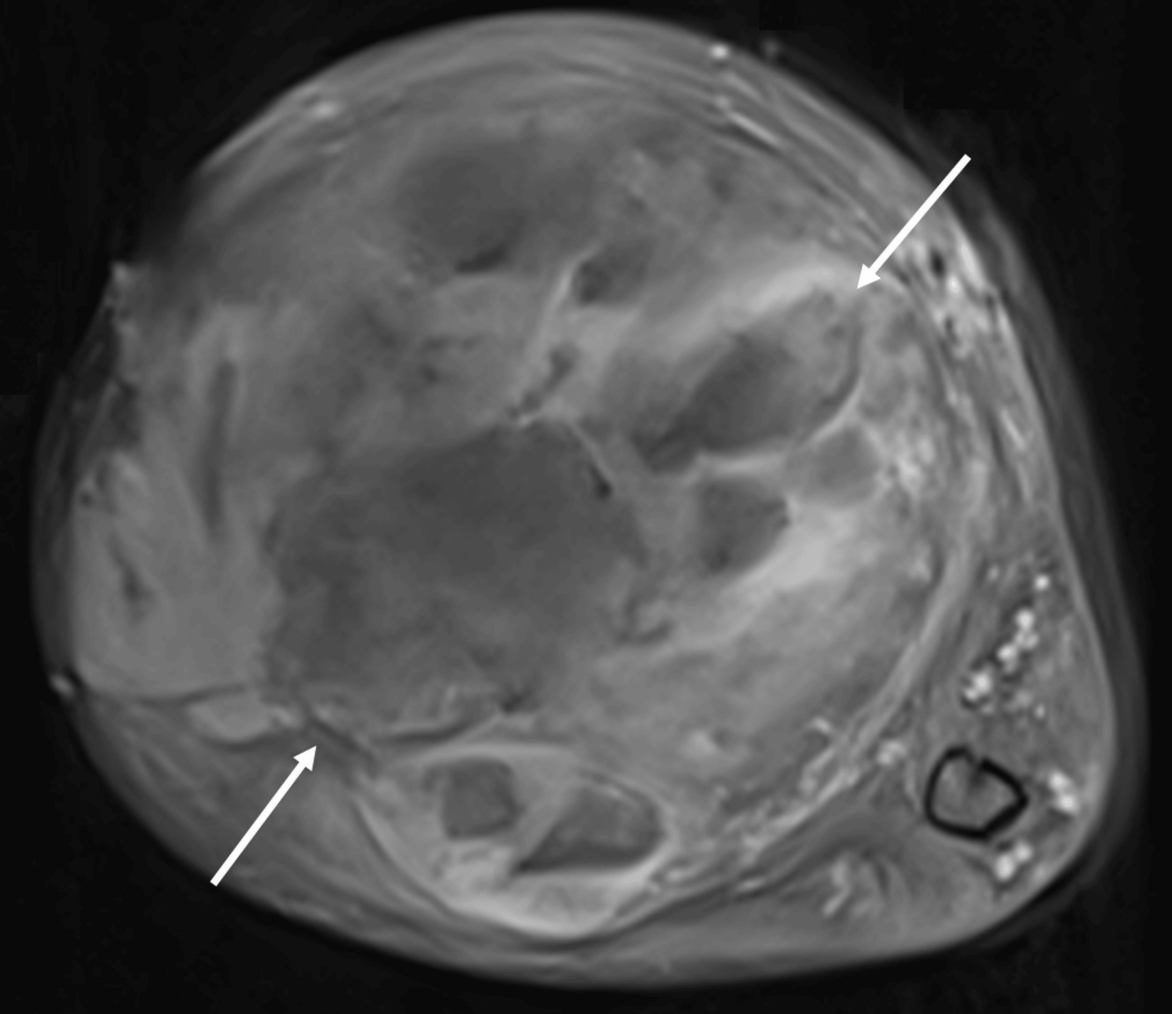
T1 axial post-contrast fat-saturated MRI of the upper arm demonstrates a heterogeneous soft tissue mass with sites of enhancement noted.
